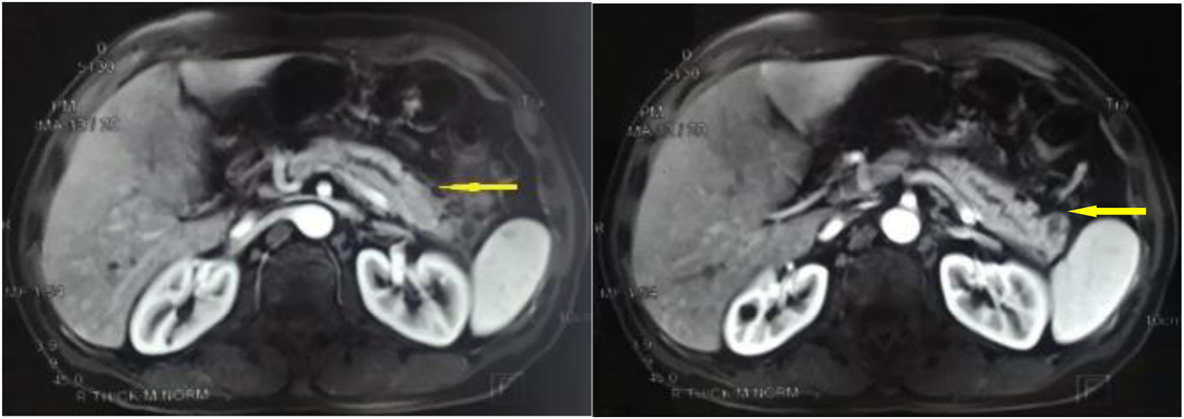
CT scan of the abdomen revealed a pancreatic space-occupying lesion and pancreatic duct dilatation
